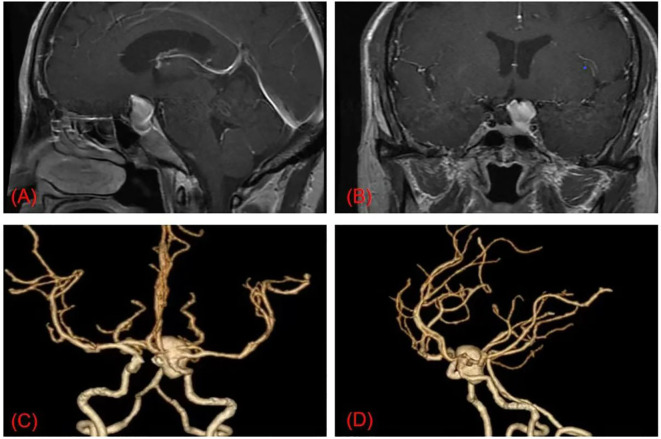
(A, B) T2-weighted magnetic resonance images of the pituitary gland, demonstrating an abnormally enhancing signal within the sellar region. This lesion is immediately adjacent to the left internal carotid artery, with no discernible plane of cleavage between them on imaging. (C, D) Cranial computed tomographic angiography (CTA) established the diagnosis of an aneurysm arising from the communicating segment of the left internal carotid artery, with the dome projecting into the sella turcica.
